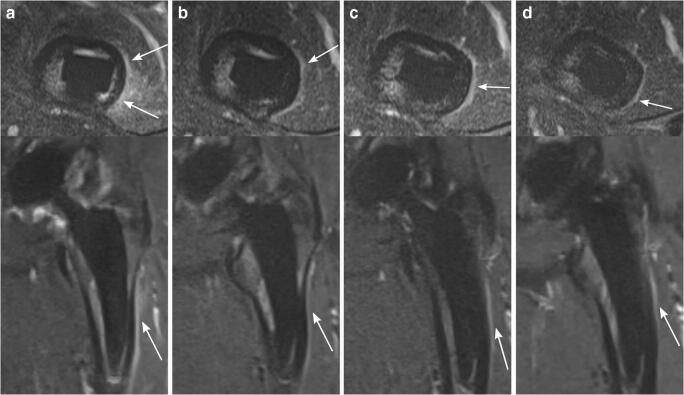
Periosteal edema on axial STIR MRI (upper row) and coronal STIR CS-SEMAC MRI (lower row). A 60-year-old asymptomatic woman with left-sided THA. Periosteal edema is present along the lateral side of the femoral shaft, accentuated in Gruen zone 2 (arrows), which persists over time. a = 3 months, b = 6 months, c = 12 months, and d = 24 months. *CS*, compressed sensing; *SEMAC*, slice encoding for metal artifact correction; *THA*, total hip arthroplasty
